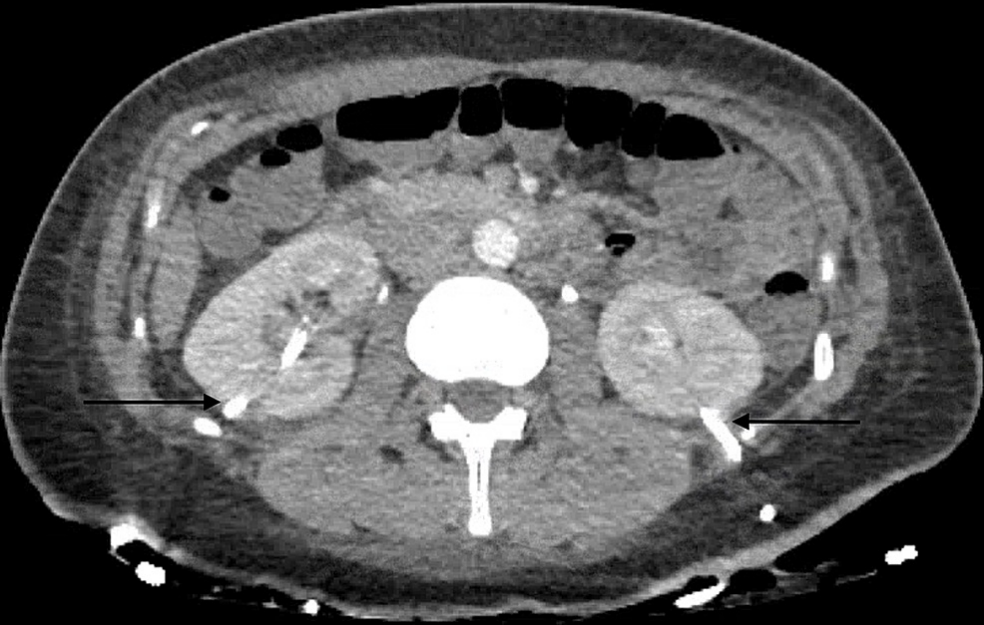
Axial view of CT urogram showing no hydronpehrosis while having the nephrostomoies clamped (left and right arrow)
